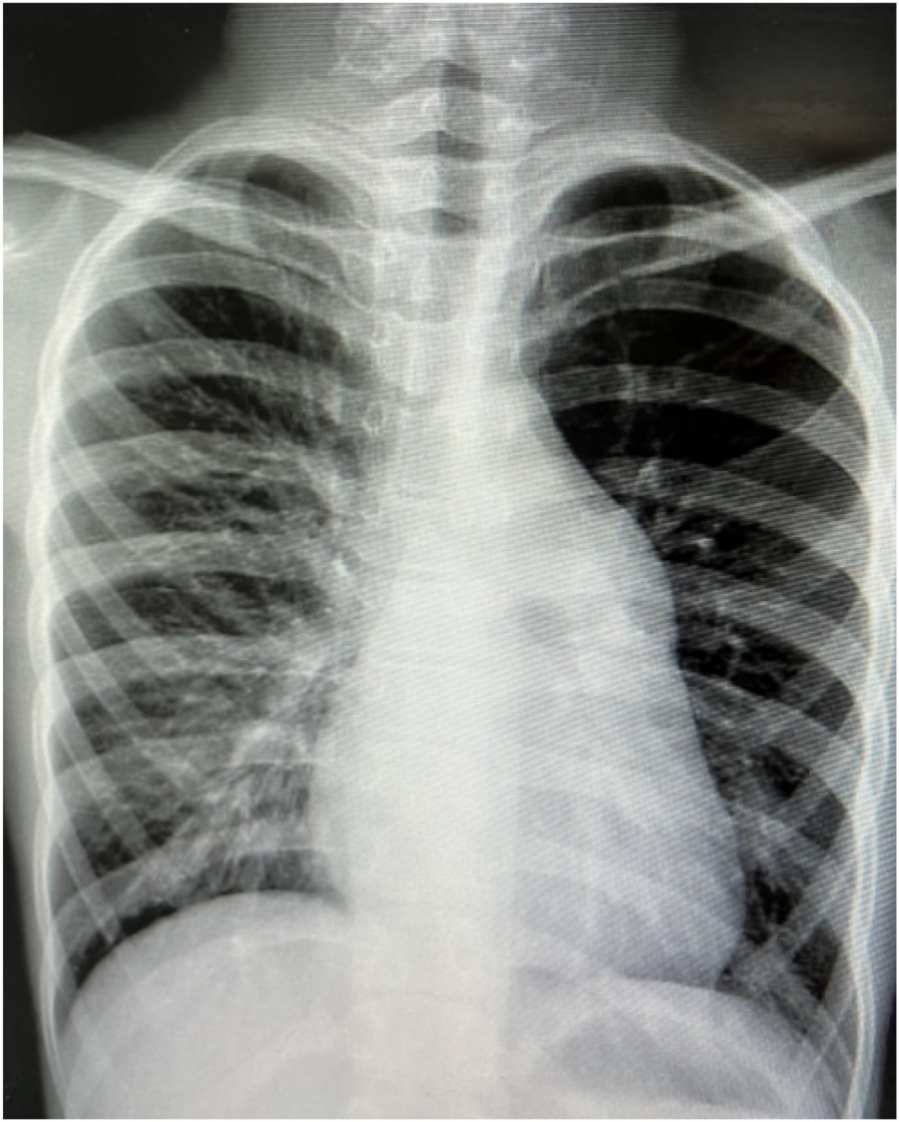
Chest x-ray of case 1 (January 11, 2024).
